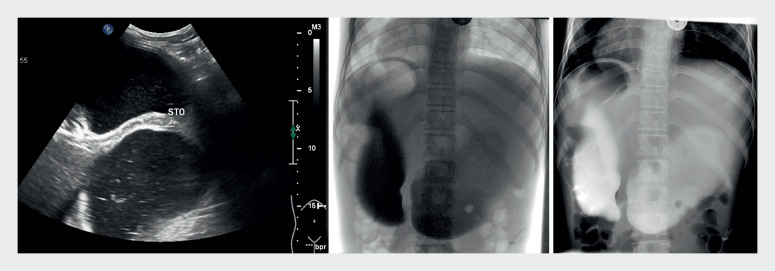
Abdominal ultrasound and X-ray “double-bubble sign.”
